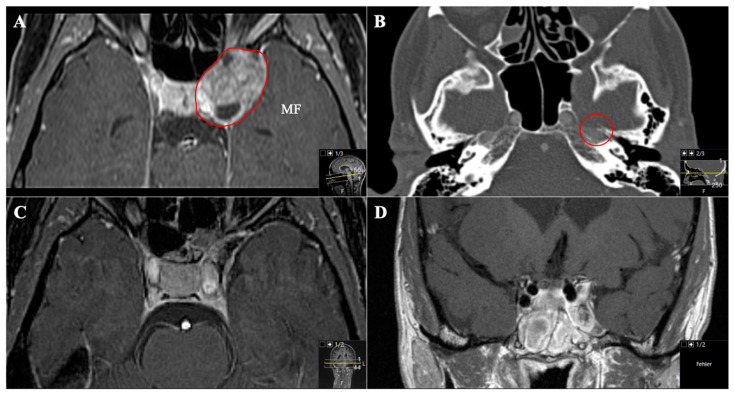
A 45-year-old female patient presented with left-sided visual impairment and headache. (A) Preoperative axial T1-weighted gadolinium-enhanced MRI and (B) axial CT, showing an optic-canal-compressing trigeminal schwannoma with a cystic part in Meckel’s cave in the middle fossa (MF, Samii Type A). Note how the foramen rotundum is widened (red circle). (C) Postoperative axial and (D) coronal MRI control, indicating complete resection via a transnasal endoscopic (transpterygoid) approach using a nasoseptal flap.
